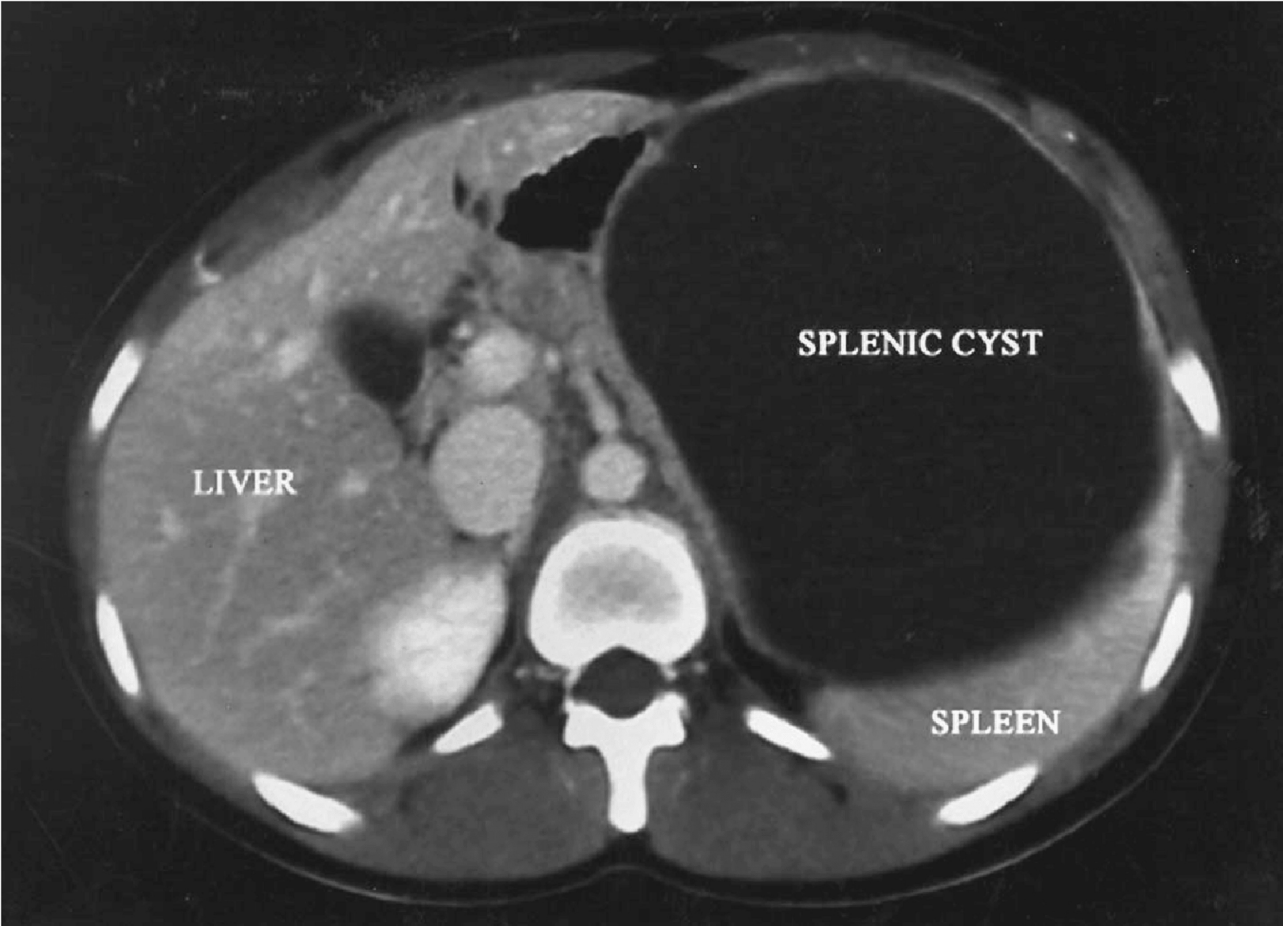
Computed tomography of the abdomen showing a 16 cm diameter fluid-filled epithelial splenic cyst in the spleen. Used with permission from Palmieri et al. [3]
